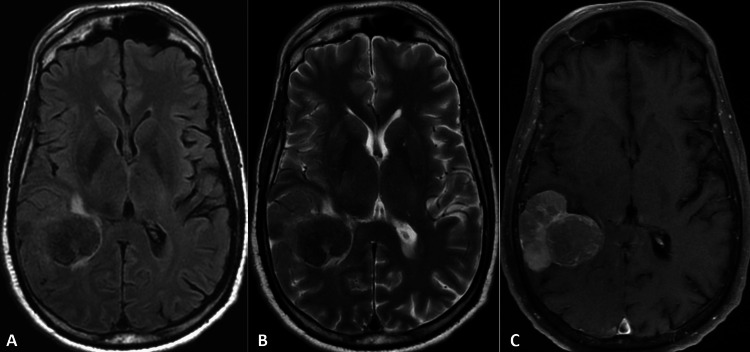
3T MRI demonstrates a heterogeneously enhancing lobulated extra-axial mass with minimal adjacent vasogenic edema, prominent flow voids, and decreased T2 signal. (A) FLAIR, (B) T2-weighted, and (C) postcontrast T1-weighted MRI: magnetic resonance imaging; FLAIR: fluid-attenuated inversion recovery
